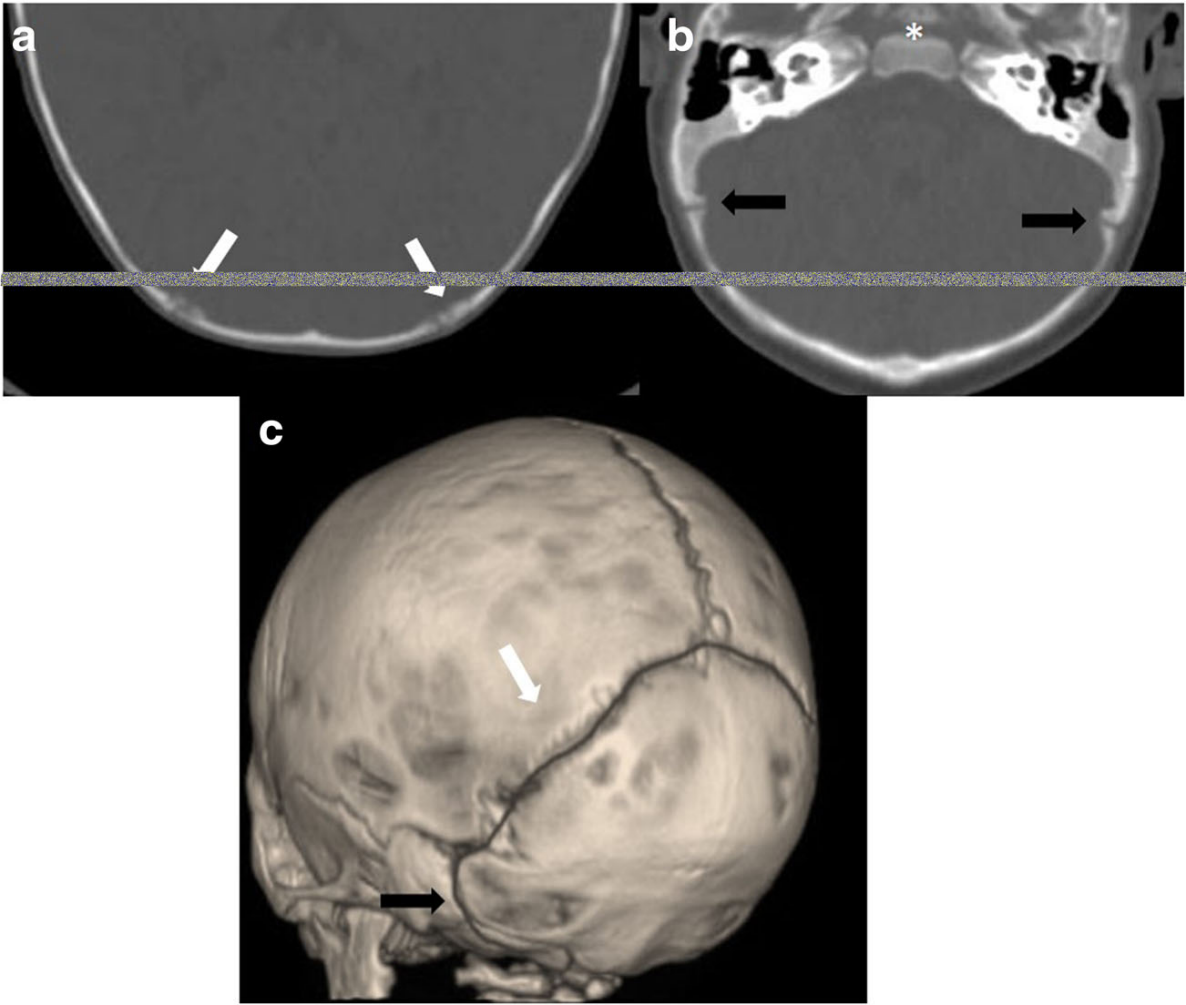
The lambdoid arch on 2D (a, b) and 3D CT scan (c) of the skull. Both the lambdoid (white arrows) and the occipito-mastoid sutures (black arrows) as well as the spheno-occipital synchondrosis (asterisk) can be appreciated
